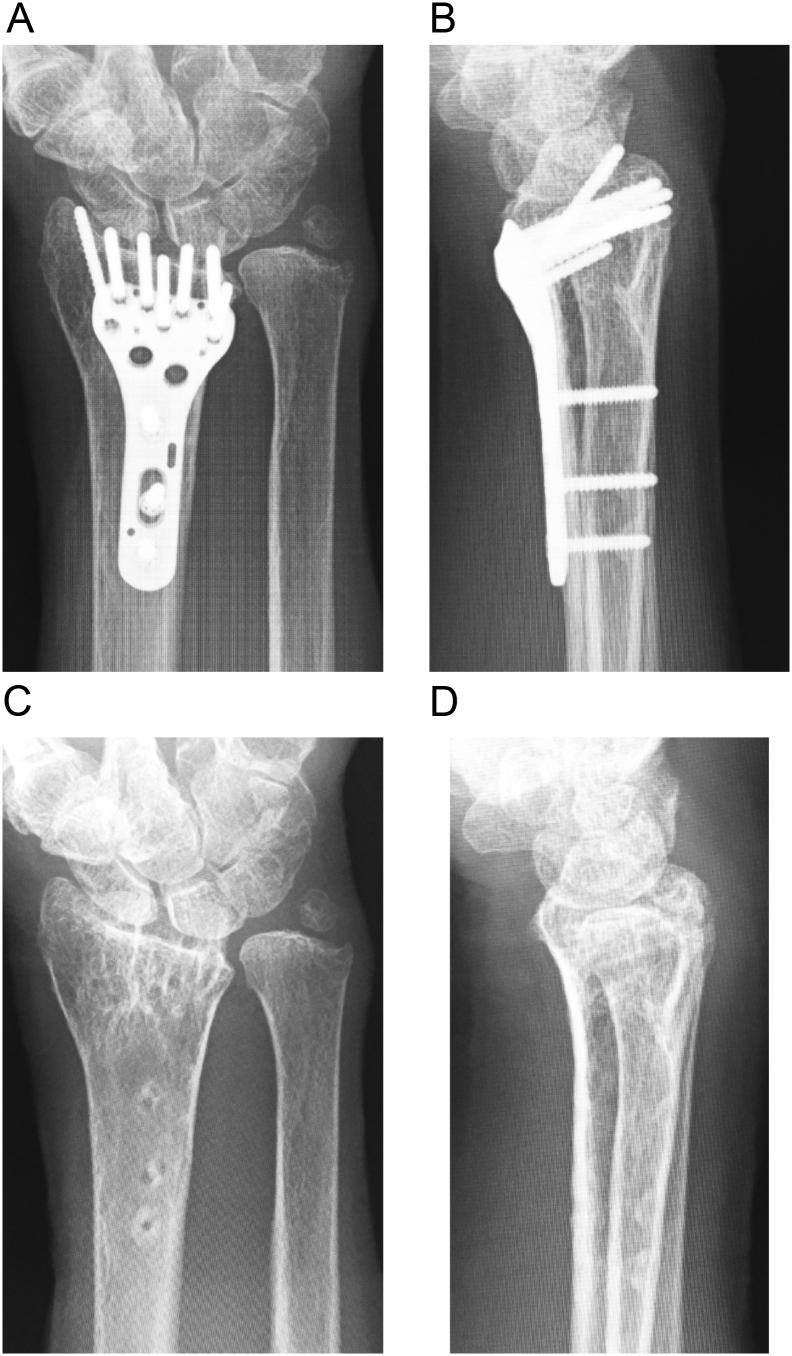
Radiographs of the distal radius at 7 years after fracture repair. A and B: preoperative images, C and D: after hardware removal.
